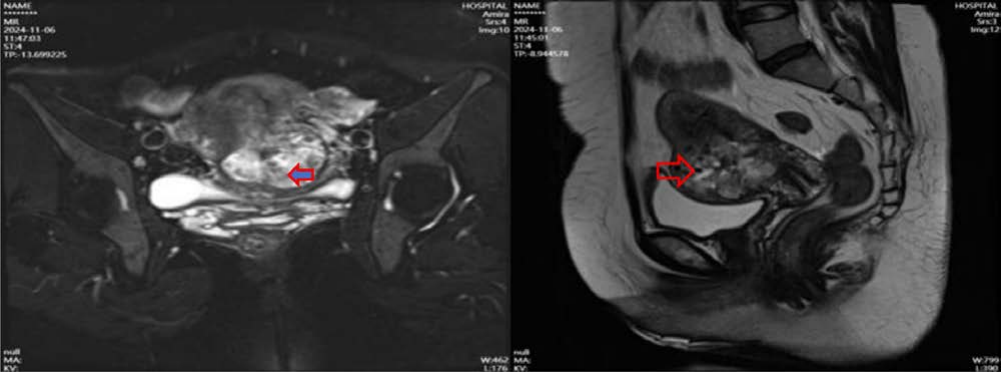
Pelvic MRI showing a mass in the lower anterior uterine wall protruding with a thin muscular layer. MRI = magnetic resonance imaging.
